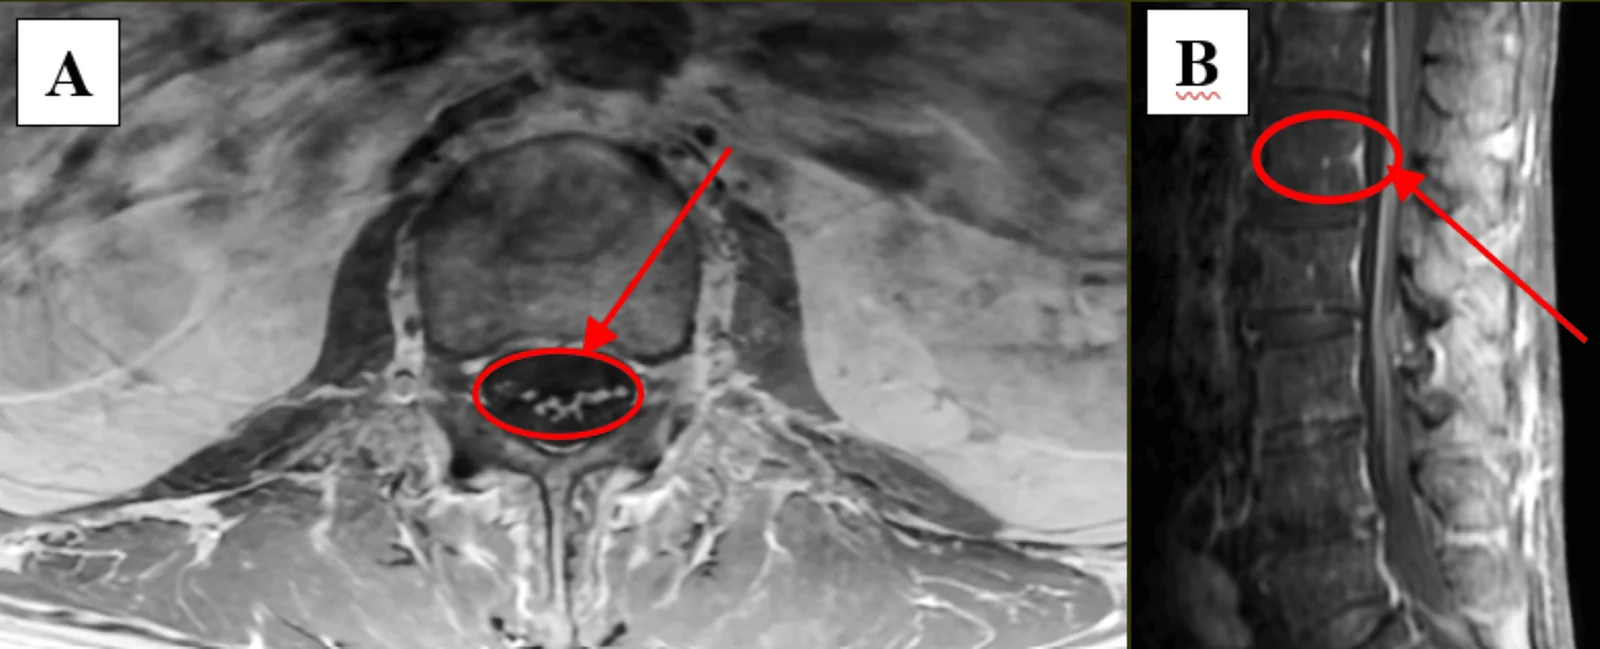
MRI findings suggestive of cauda equina nerve root involvement (A) Contrast-enhanced axial T1-weighted MRI of the lumbar spine demonstrates smooth enhancement of the cauda equina nerve roots, indicated by the arrow. (B) Sagittal lumbar spine MRI similarly demonstrates abnormal signal/enhancement along the cauda equina nerve roots. In the appropriate clinical context, these findings are supportive of inflammatory polyradiculoneuropathy and Guillain-Barré syndrome-like involvement.
